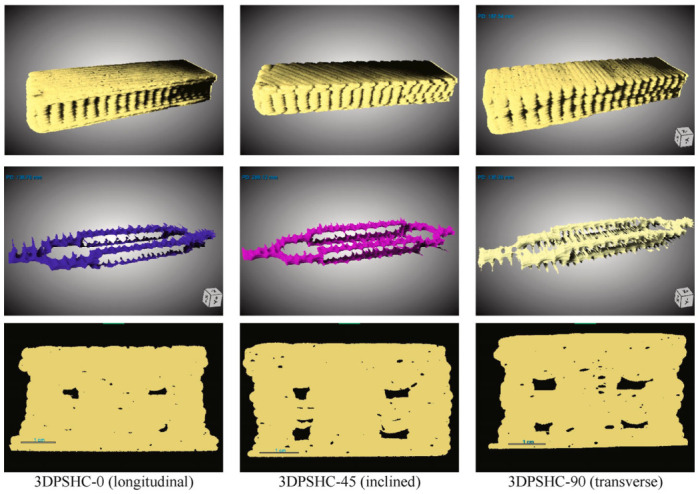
CT reconstruction of hollow vascular channels in 3D-printed cement slurry [76].
